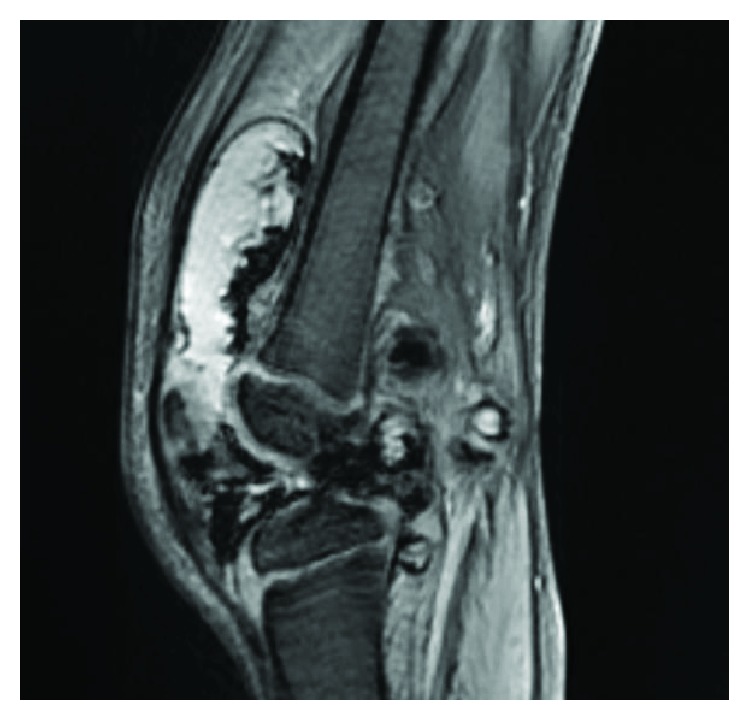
MRI, T2 sequence of the left knee: important joint effusion.
